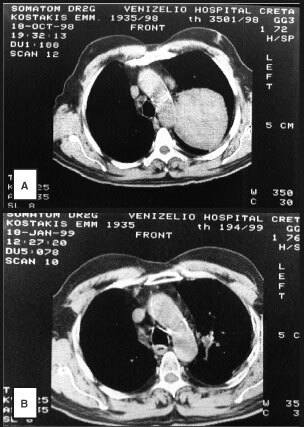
A CT-scan from a lung squamous carcinoma before (A) and after the end (B) of Taxotere®/Caelyx® chemo–radiotherapy.
